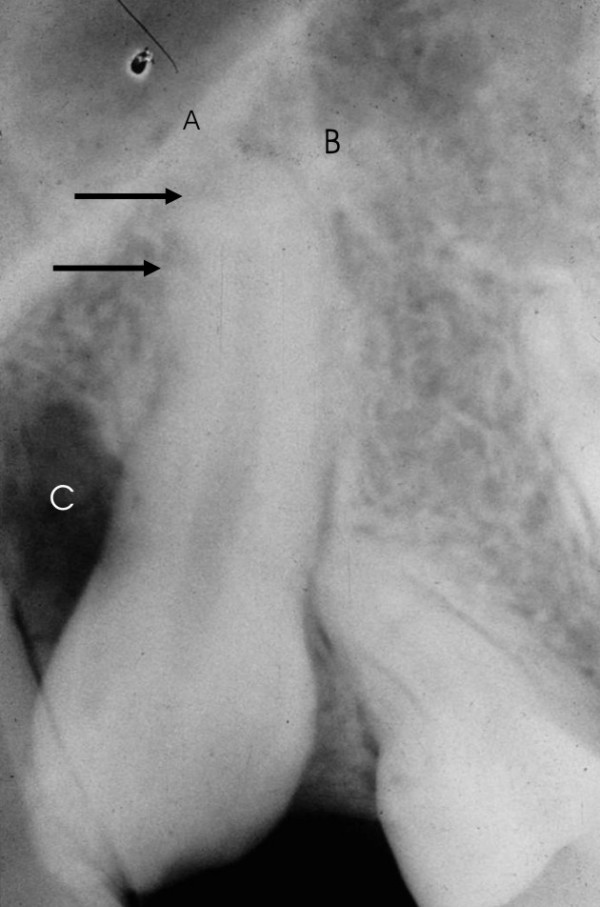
Periapical radiograph for confirmation the presence of the impacted tooth. aA. Nasal cortical; B. Sinusal cortical (both corticals compose the Ennis inverted Y), the arrow points to dilaceration with possible apical ankylosis; C. Bone loss with fibrous scar, possibly due to two previous surgeries.
